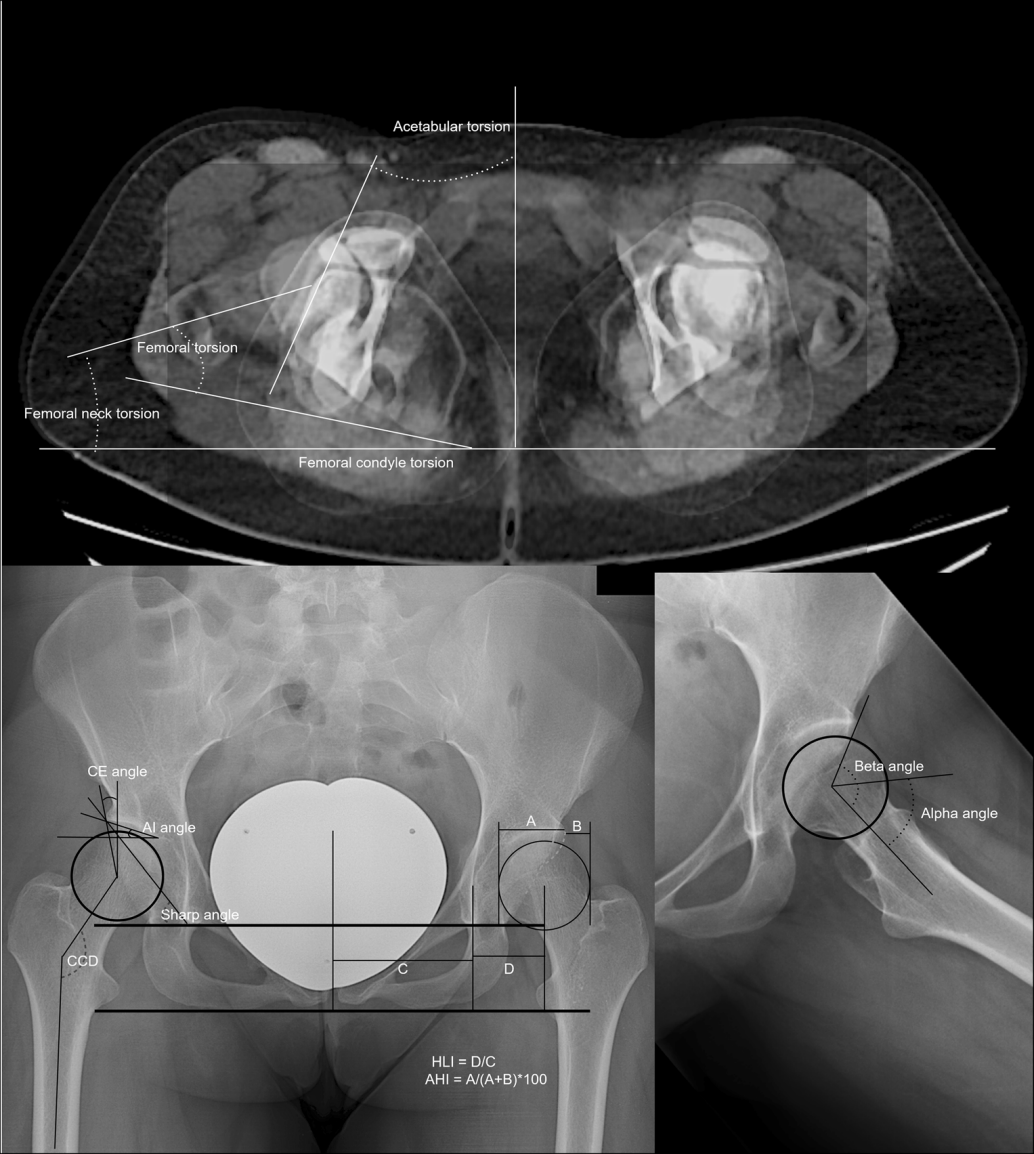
Measurements performed on plain radiograps and in rotational CT
